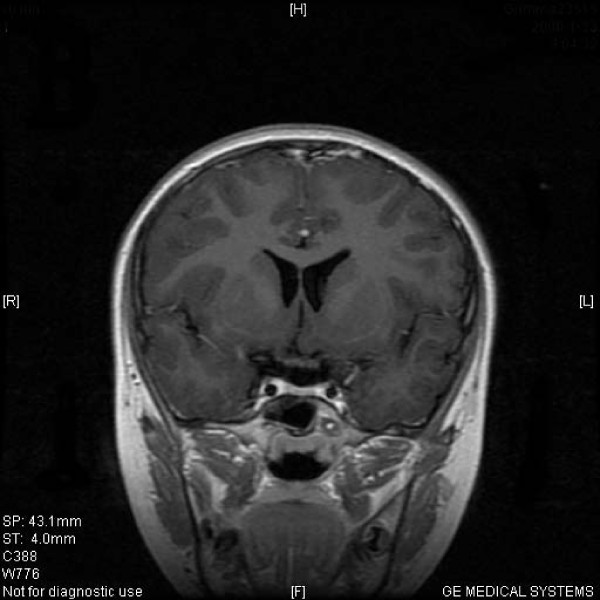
Typical MRI scan changes in GH adenoma. No significantly enhancing mass lesion is seen in the sella turcia under the T1-weighted postcontrast MRI scan performed 1 year after the second MASEP GKRS. Patient 3's clinical symptom did improve. His serum growth hormone level was lower than 10 ng/ml.
